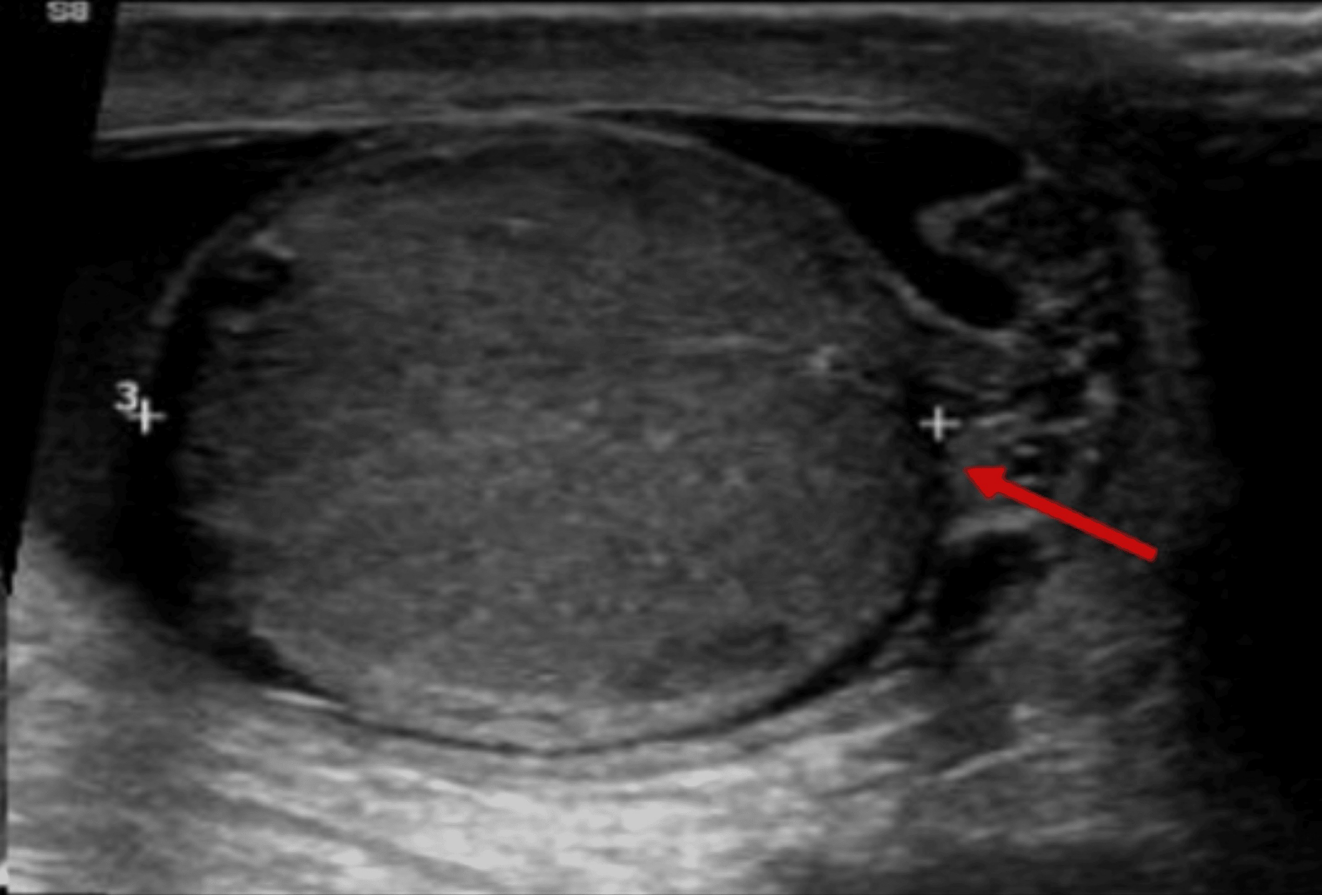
Ultrasound of scrotum showing discontinuity of tunica albuginea (arrow) at the inferior pole of the left testis.
